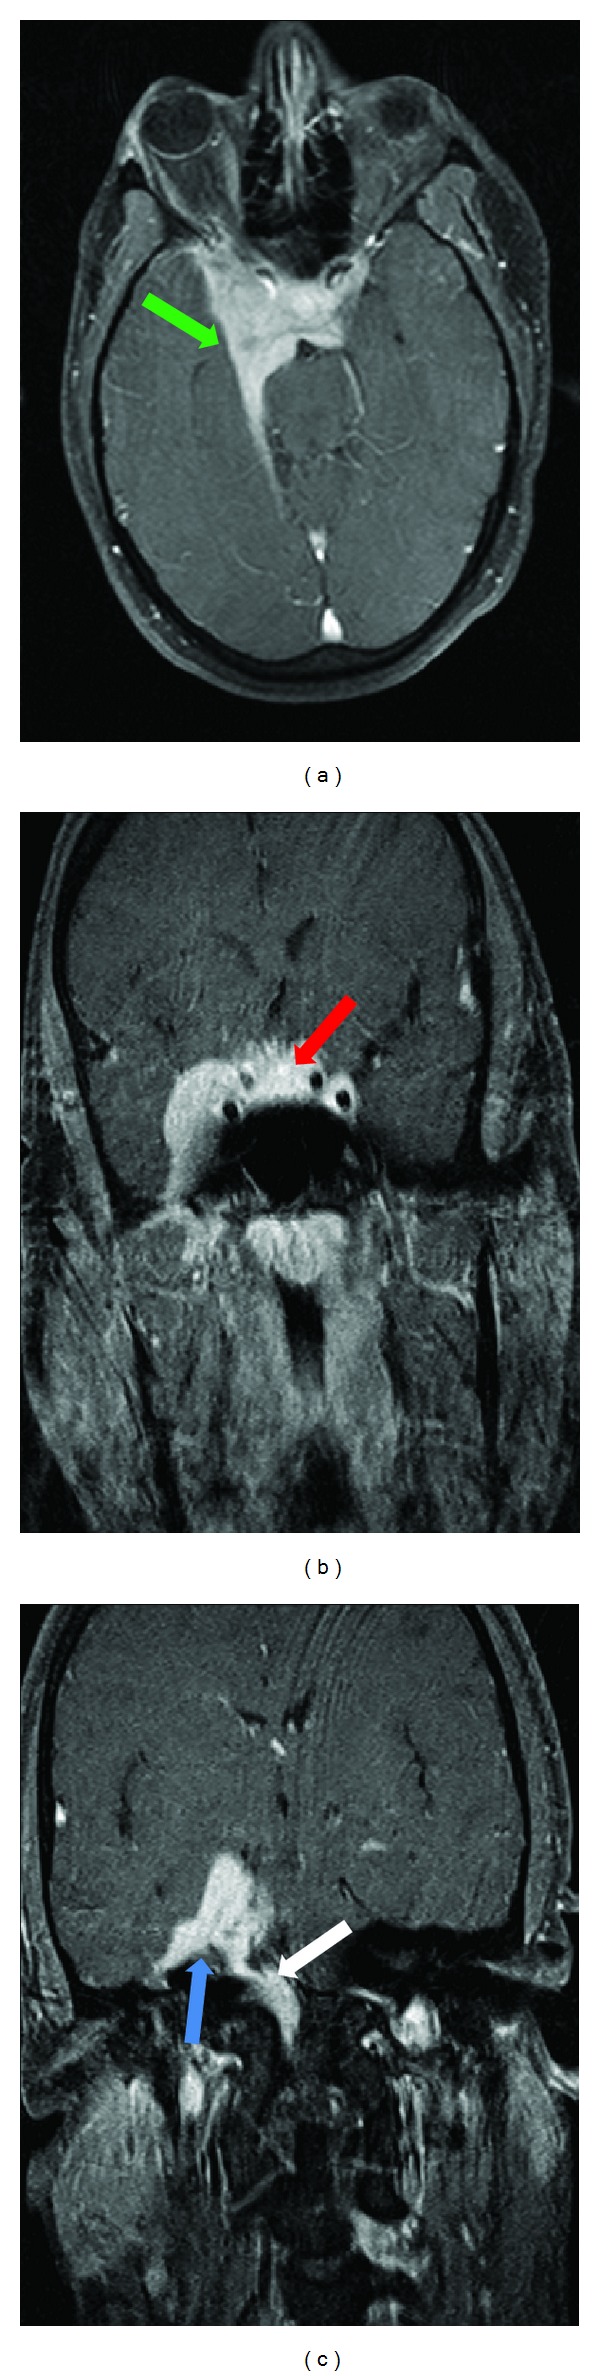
Axial (a) and coronal (b, c) T1-weighted fat suppressed images following administration of 15 mL intravenous gadolinium. There is diffuse enhancement within the skull base (green arrow), cavernous sinus (red arrow), internal auditory canal (blue arrow), prepontine cistern, and inferior extension of the enhancing lesion (white arrow) into the brainstem characteristic of granulomatous changes in neurosarcoidosis.
